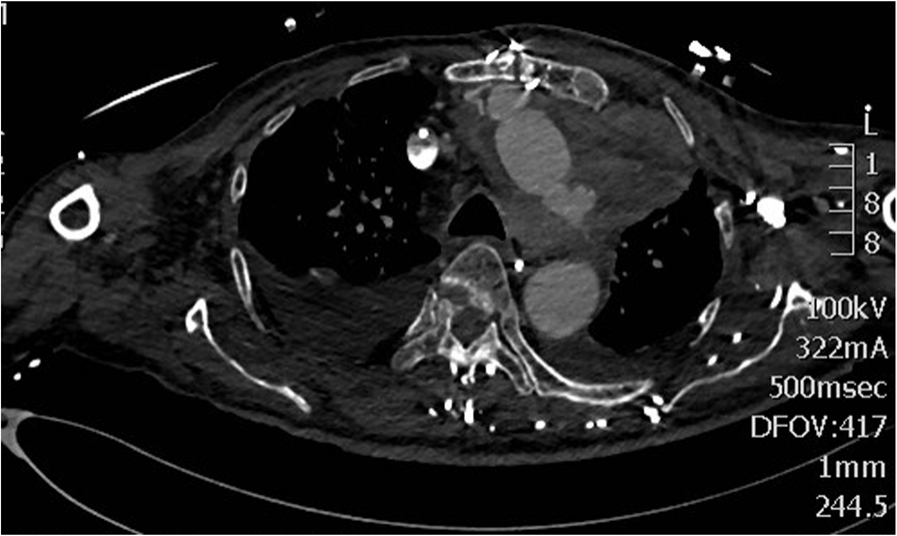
CT scan showing large leak with huge hematoma around proximal descending aorta
